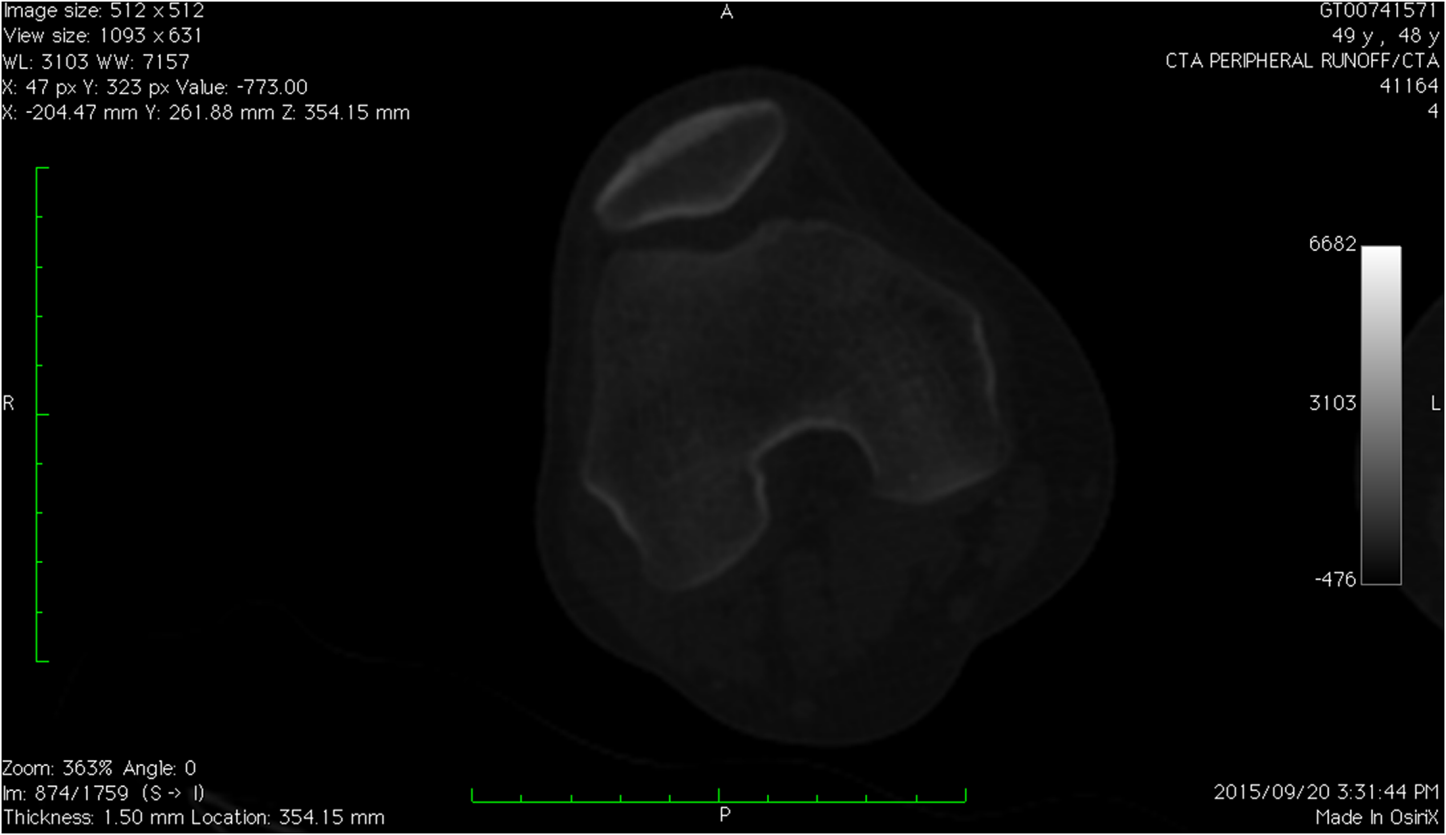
CT image of a distal femur of a living human—fresh bone. The cortex of the bone appears white with the mineralized matrix also appearing as shades of white to grey. Basic iterative reconstruction technique. Scale = 10 cm total—each bar = 1 cm.
